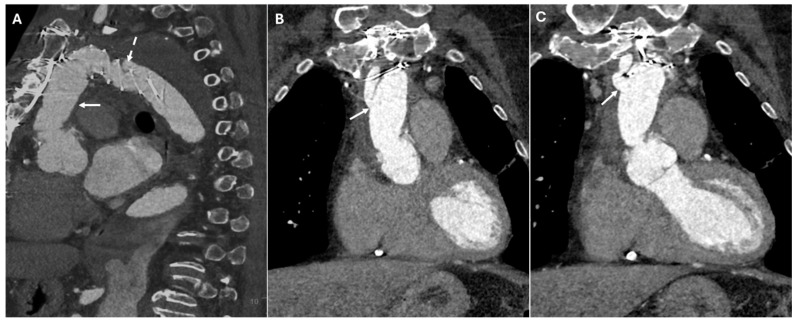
CT images of the frozen elephant trunk. These images provide a comprehensive view of the frozen elephant trunk procedure, which combines open surgical and endovascular techniques to treat extensive thoracic aortic disease, particularly in cases involving the aortic arch and descending aorta. Panel (A) shows the open proximal graft (white arrow) and the distally extended stent (dashed line). Panels (B,C) depict the arch vessels connected to the aortic graft (white arrows). These demonstrate the anatomical relationships and patency of the revascularized supra-aortic branches, which are crucial for maintaining adequate cerebral and upper-extremity perfusion.
